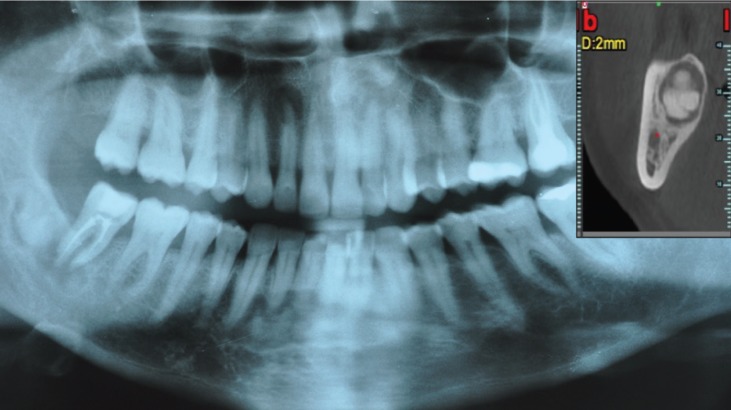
Orthopantomography 2014.
